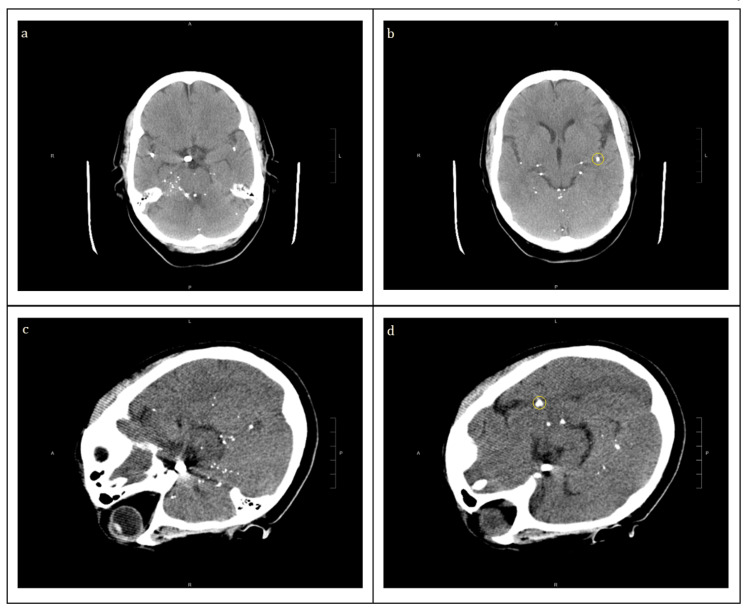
(a, b) CT brain without contrast showing multiple small round hyperdense spots in the sulci of cerebrum and cerebellum, distributing along the meninges, tentorium, and in the internal auditory canals bilaterally. (c, d) Repeat CT brain is performed with the right side of the head down for two-and-half hours, again showing innumerable hyperdense foci appearing similar in distribution; for reference, the yellow circles in (b) and (d) show the same hyperdense spot without migration.
